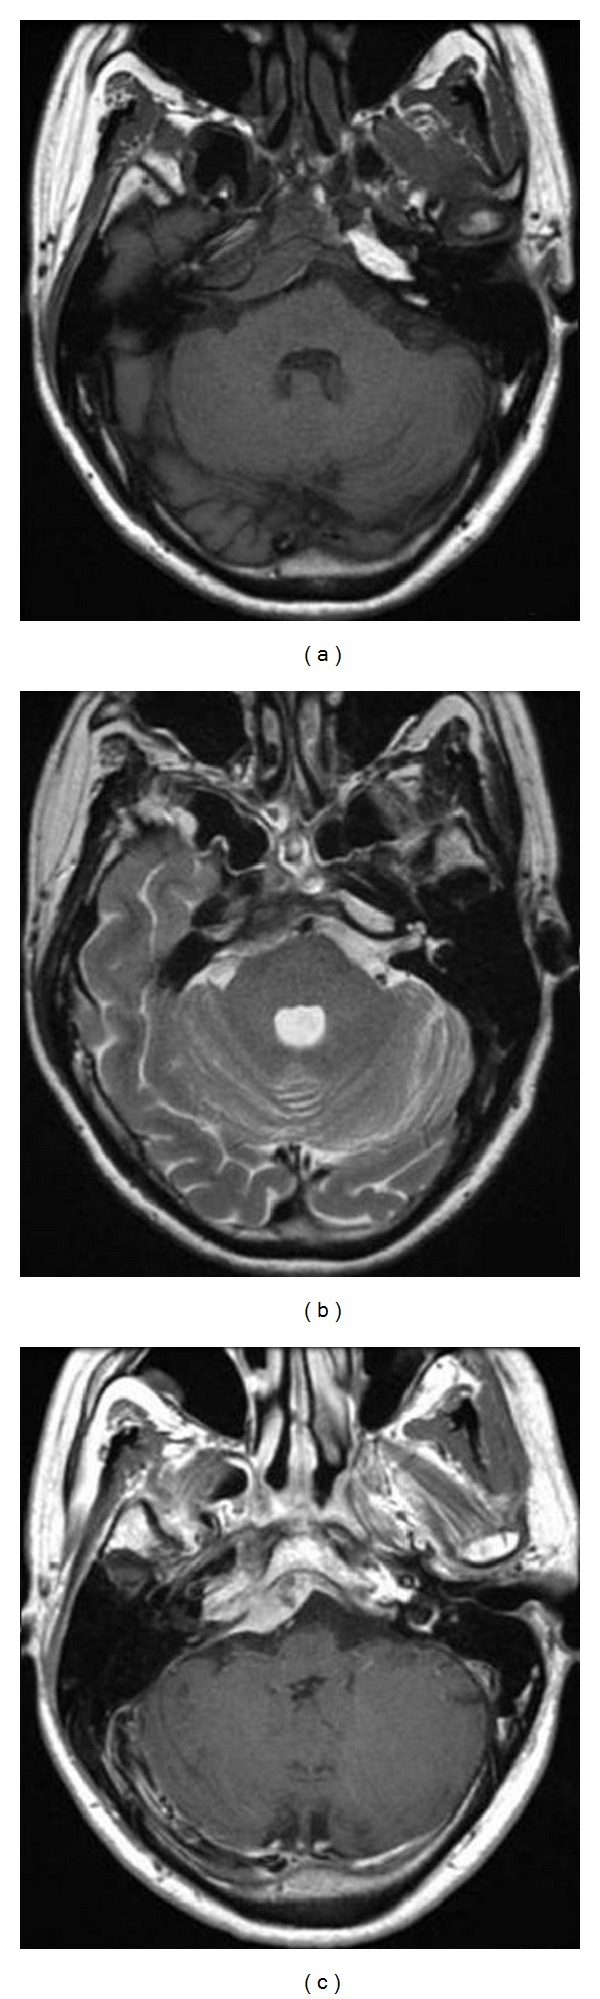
(a) Cerebral T1-weighted MRI shows a homogeneous, low-signal mass in the petrous bone from the right clivus. (b) Cerebral T2-weighted MRI reveals a homogeneous, high-signal mass in the petrous bone from the right clivus. (c) Cerebral T1-weighted MRI with gadolinium shows a heterogeneously enhanced mass in the petrous bone from the right clivus.
